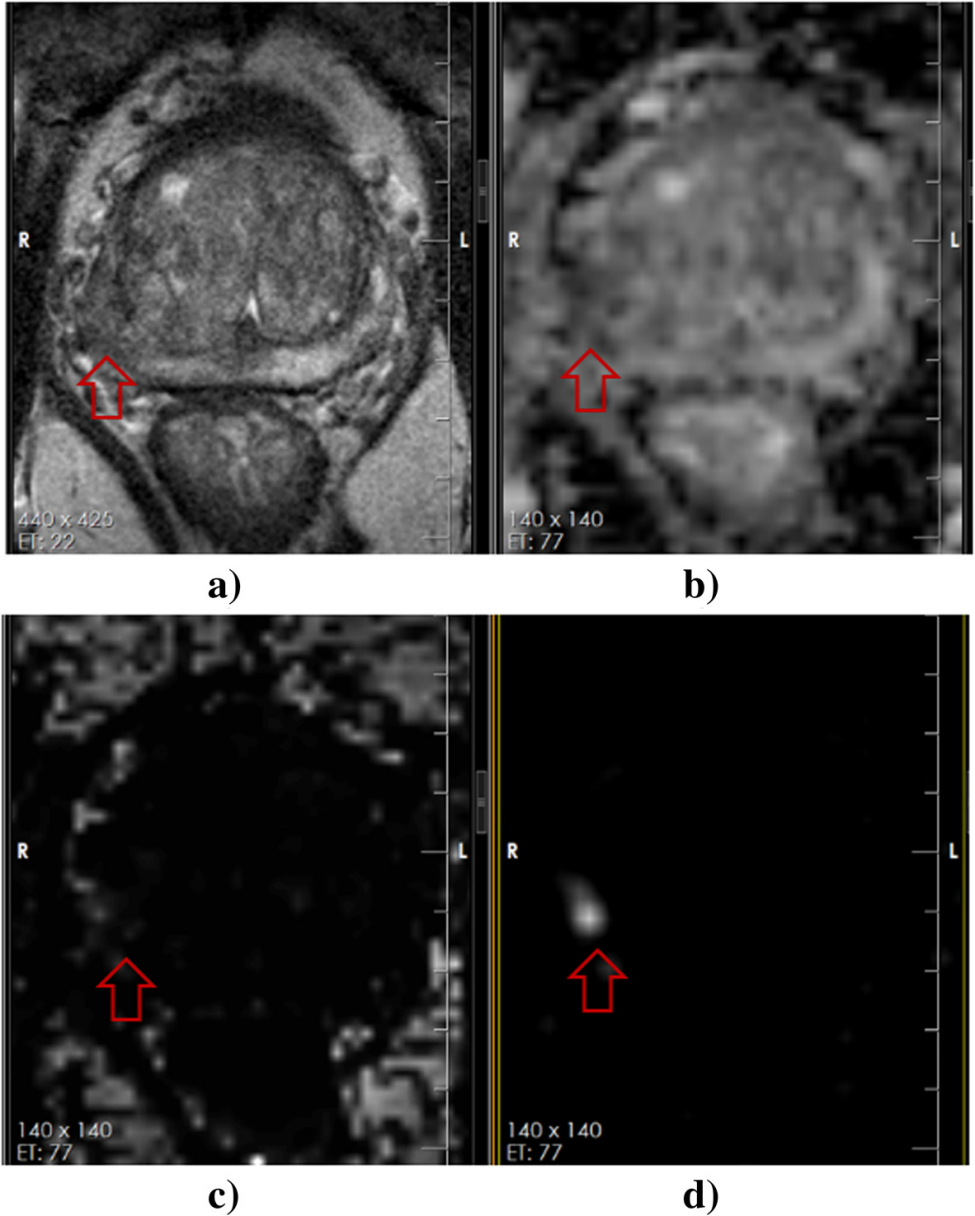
a T2w does not clearly show a tumour although there is mild signal alteration in the left peripheral zone (arrow). b ADC does not clearly show a tumour (arrow). c CHB-DWI of 2000 *s*/*m*
